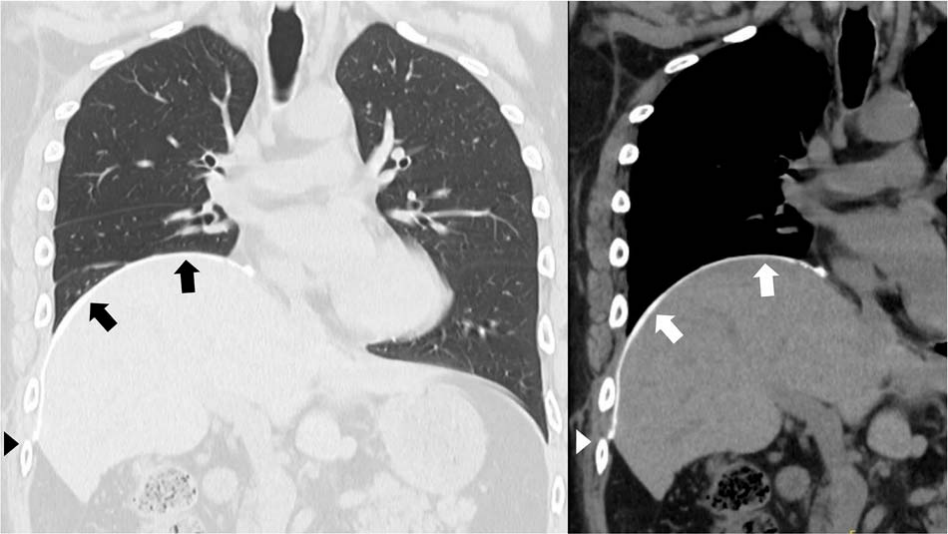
Postoperative chest computed tomography showing the reconstructed diaphragm using a second polytetrafluoroethylene (PTFE) patch above the original placement, overlaying the diaphragmatic defect that had been closed by the first PTFE patch (arrow). The second PTFE patch was fixed to the lower ribs (arrowhead).
